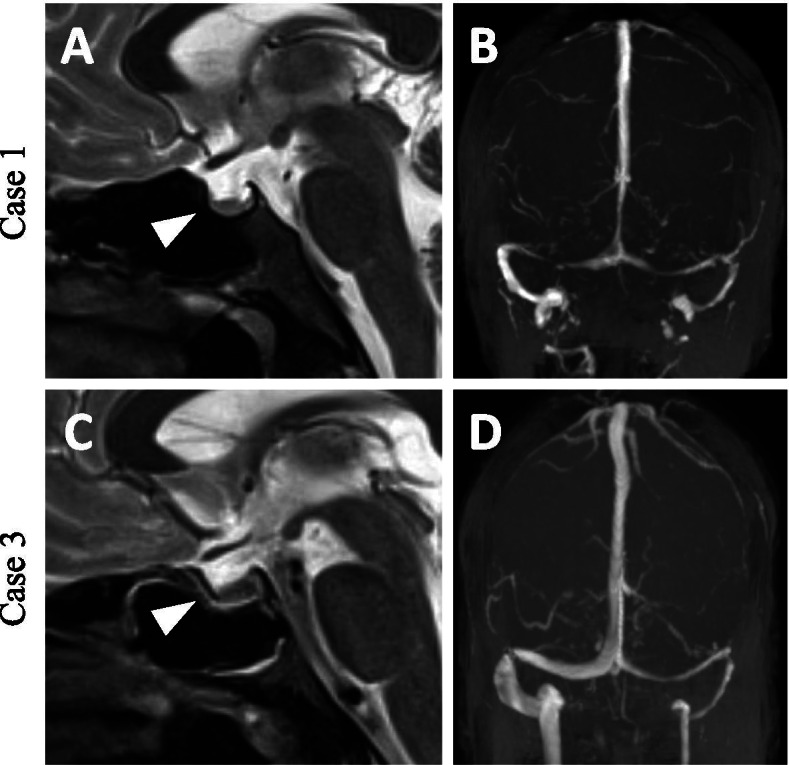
MRI scans of case 1 and 3 with typical signs of IIH. A + C Small pituitary gland also described as empty sella (white arrow heads). B + D Thin transverse sinus as an indirect sign of IIH. Note: Case 2 brought external realised MRI scan found unremarkable
